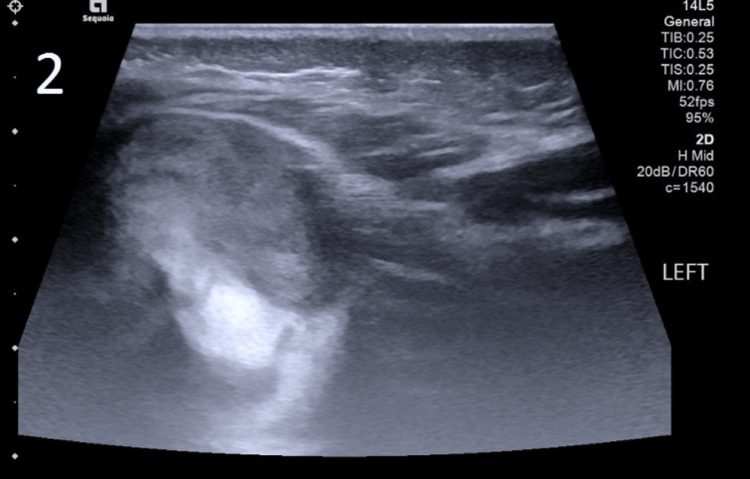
Ultrasound of the left knee joint The ultrasound revealed thickening of the synovium with mild joint effusion.
